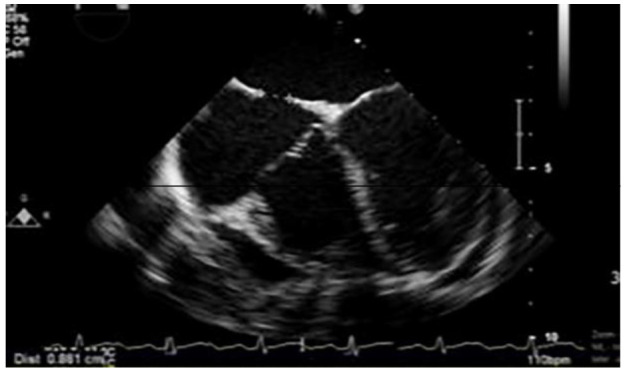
Transthoracic echocardiography in global view showed Atrial Septal Defect type 2
